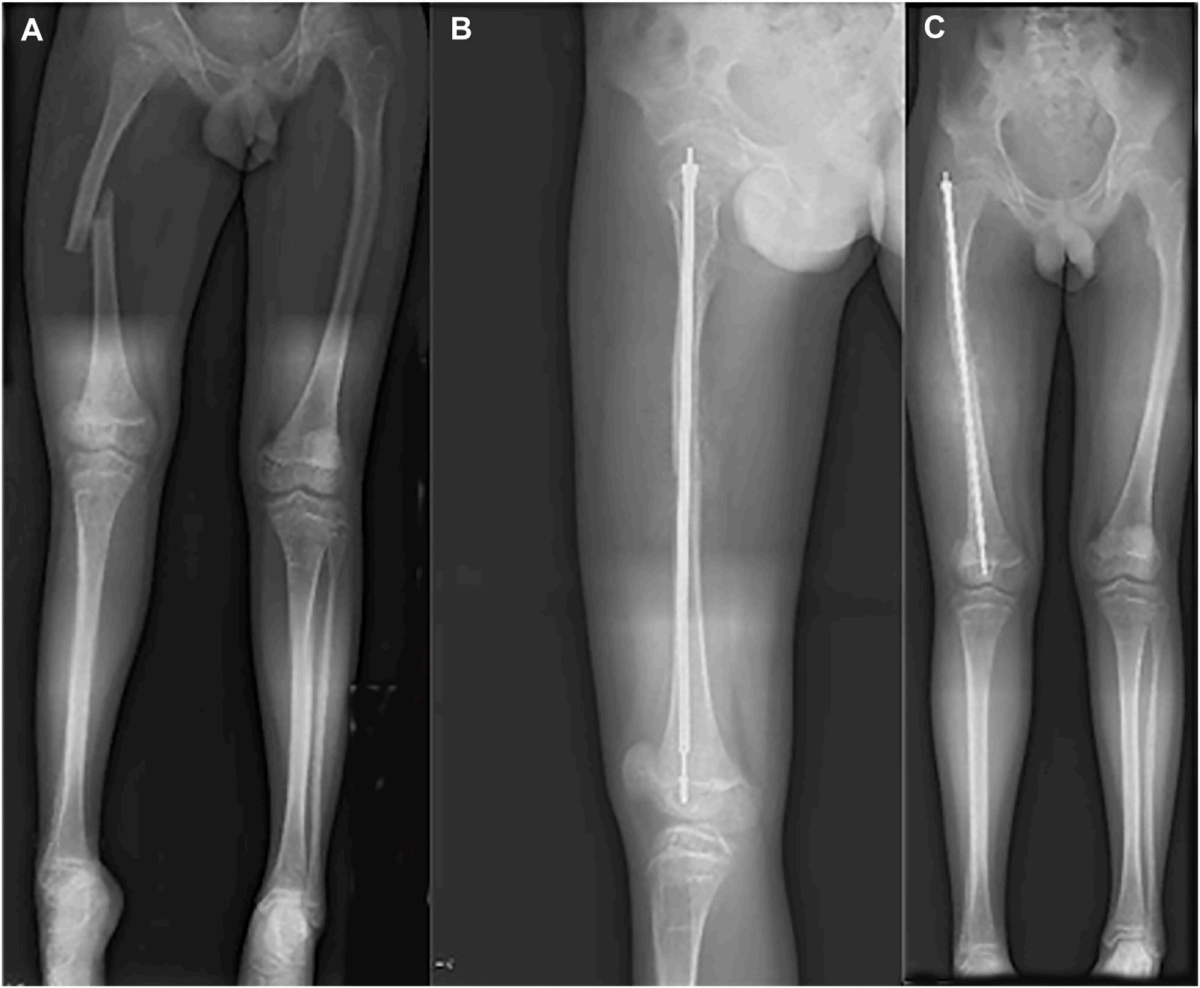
Radiograph of 13-year-old boy with right femoral fracture. (A) Full-length AP view of lower extremity before surgery. (B) Lateral of femur after surgery. (C) Full-length AP view of lower extremity after surgery.
